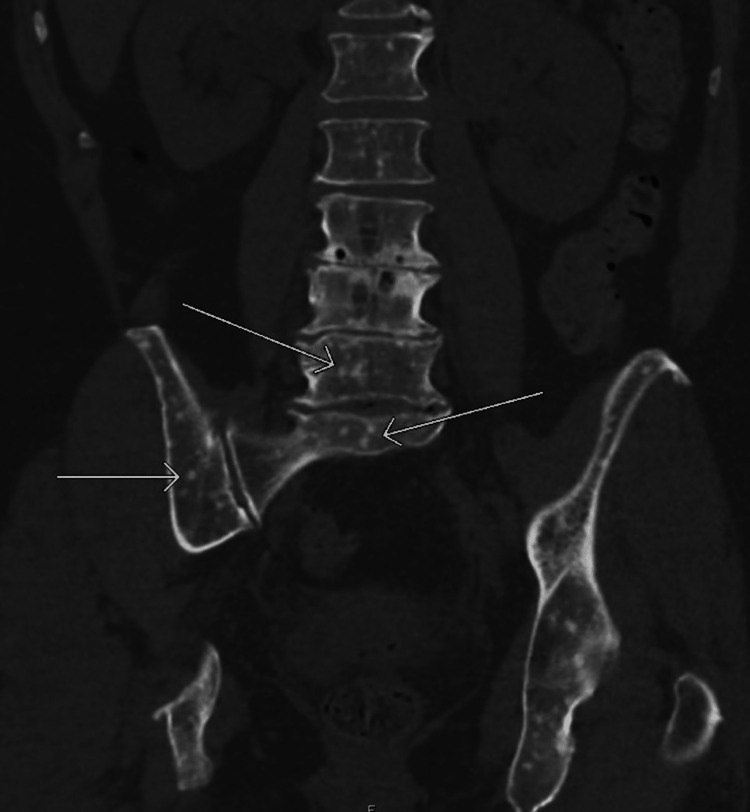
Coronal view of CT renal scan without signs of urolithiasis. Evidence of diffuse osseous lesions suspicious for metastasis throughout the axial skeleton (white arrows).
